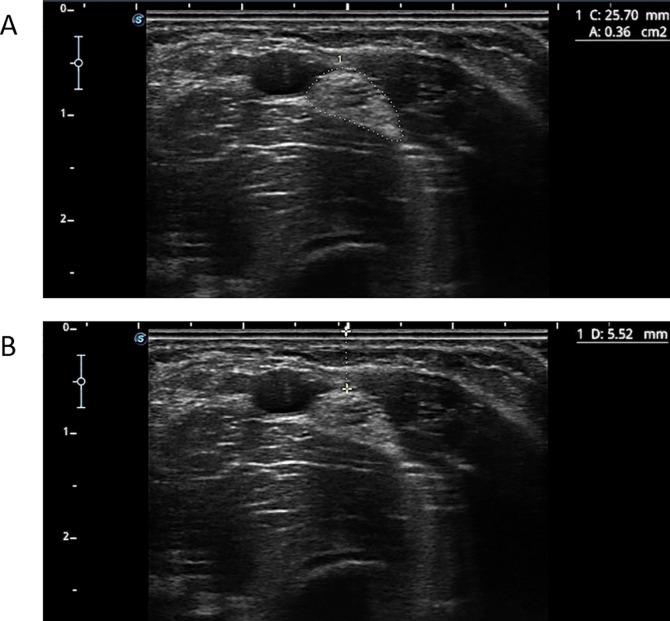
Ultrasound image of the MN where proximal measurements were performed. The cross-sectional area (CSA) (A) and the nerve-to-skin distance (B) are shown.
